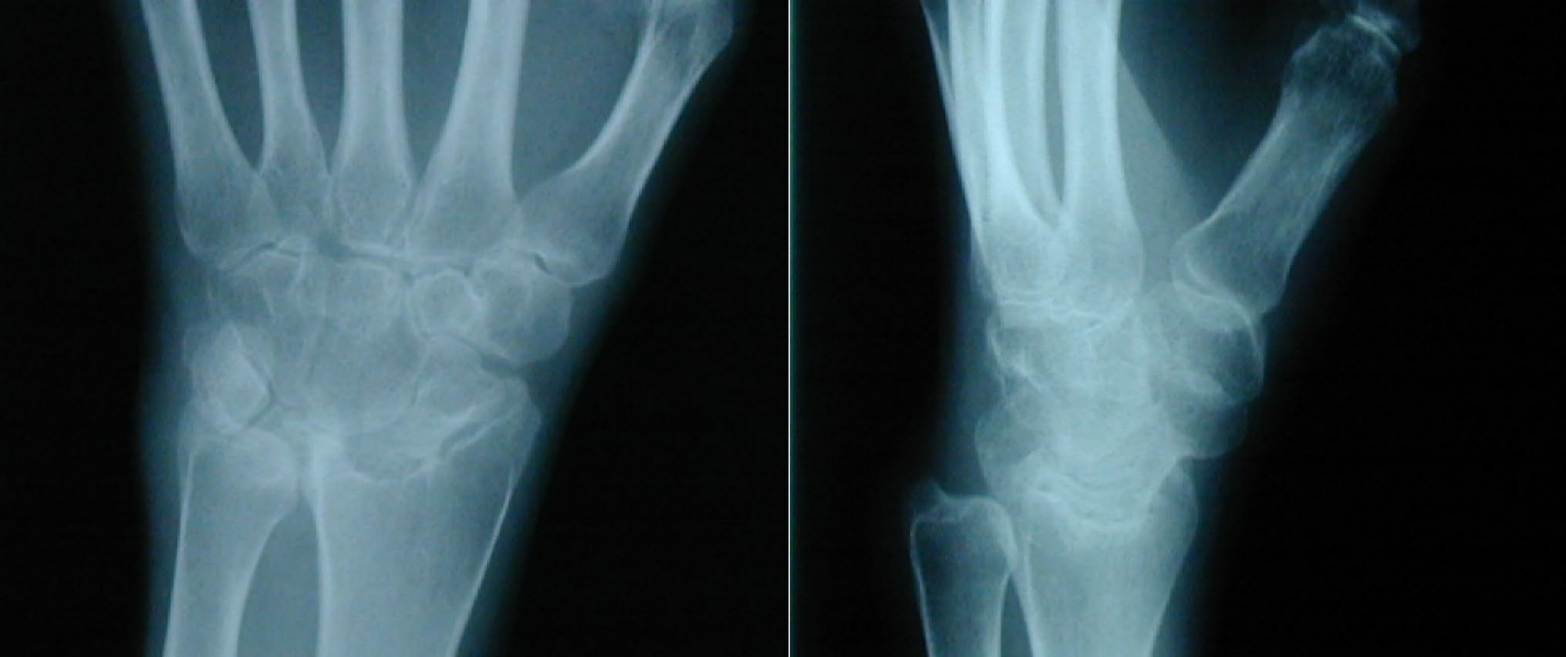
Case 2 - Left preoperative X-ray in lateral and a.p. view
